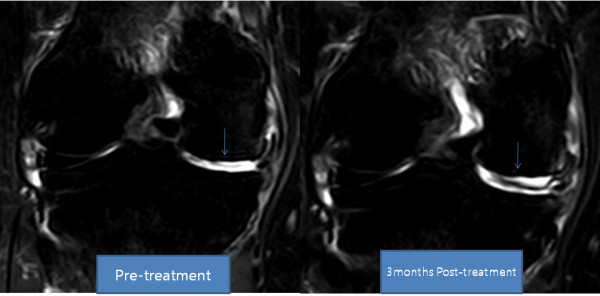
MRI coronal T2 view of the knee. Pre-treatment and post-treatment MRI shows increased height of medial meniscus (arrow).
